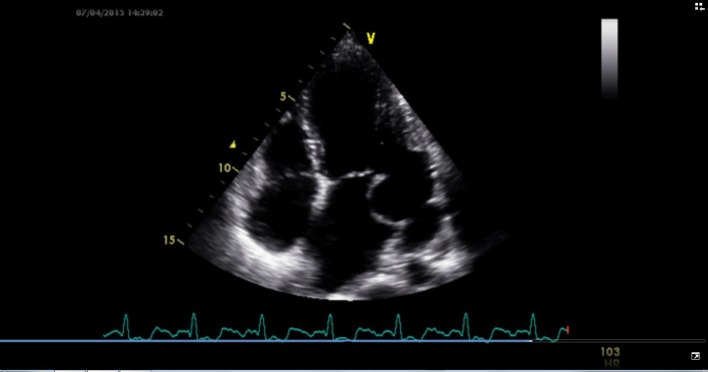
Transthoracic echocardiography in apical four-chamber view showing submitral aneurysm in the posterolateral wall of the left ventricle.
